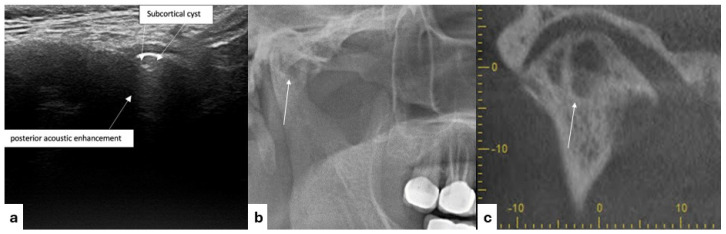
Ultrasonographic (a) and radiographic images of a subcortical cyst (b,c).
